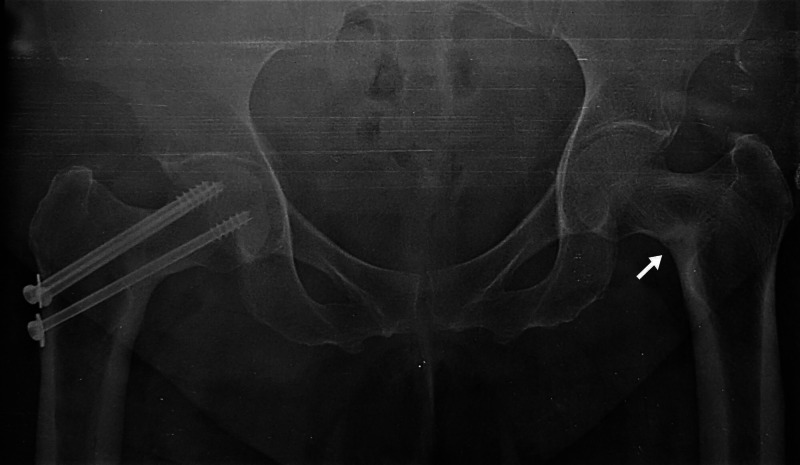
Anteroposterior both hip radiograph with right femoral neck fracture showing no avascular necrosis or collapse, bilateral varus angulation of femoral neck with sclerosis along inferomedial aspect of left neck femur (white arrow).
